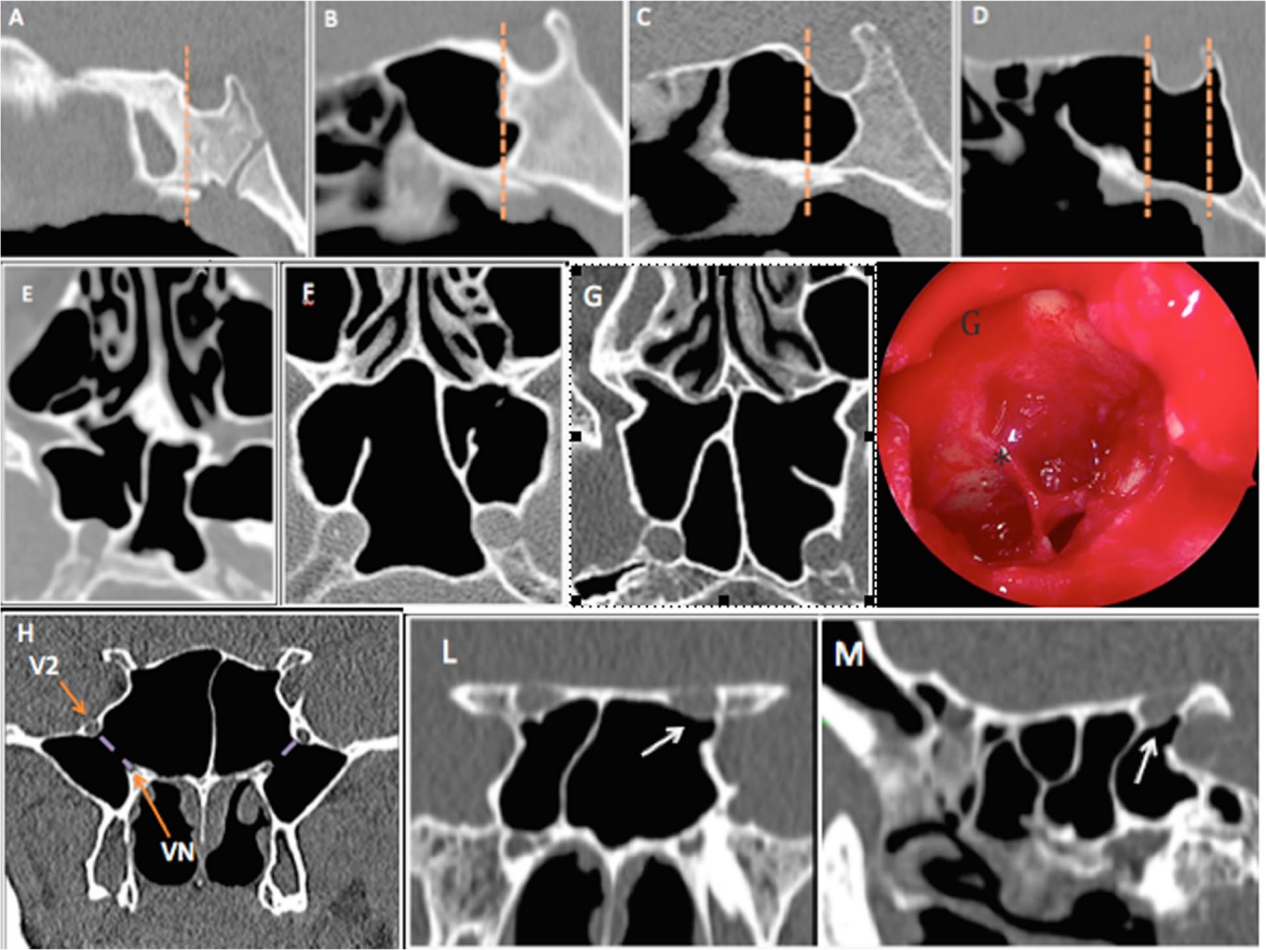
Güldner’s classification^7^ of sphenoid sinus pneumatization on sagittal CT images: A conchal, B presellar, C sellar, and D postsellar pneumatization types. Axial CT images showing (E) right dehiscent internal carotid artery (ICA) and protrusion of accessory sphenoid septa into the left ICA, F deviation of the sphenoid septum in left ICA protrusion, G accessory septations with attachment on the right ICA; G endoscopic right sphenoid sinus show an accessory septations (*). Coronal (H) CT image revealing bilateral pterygoid recess pneumatization. Coronal (L) and sagittal (M) CT scans showing dehiscence of the left optic nerve (ON) and attachment onto the sphenoid sinus (white arrow)
